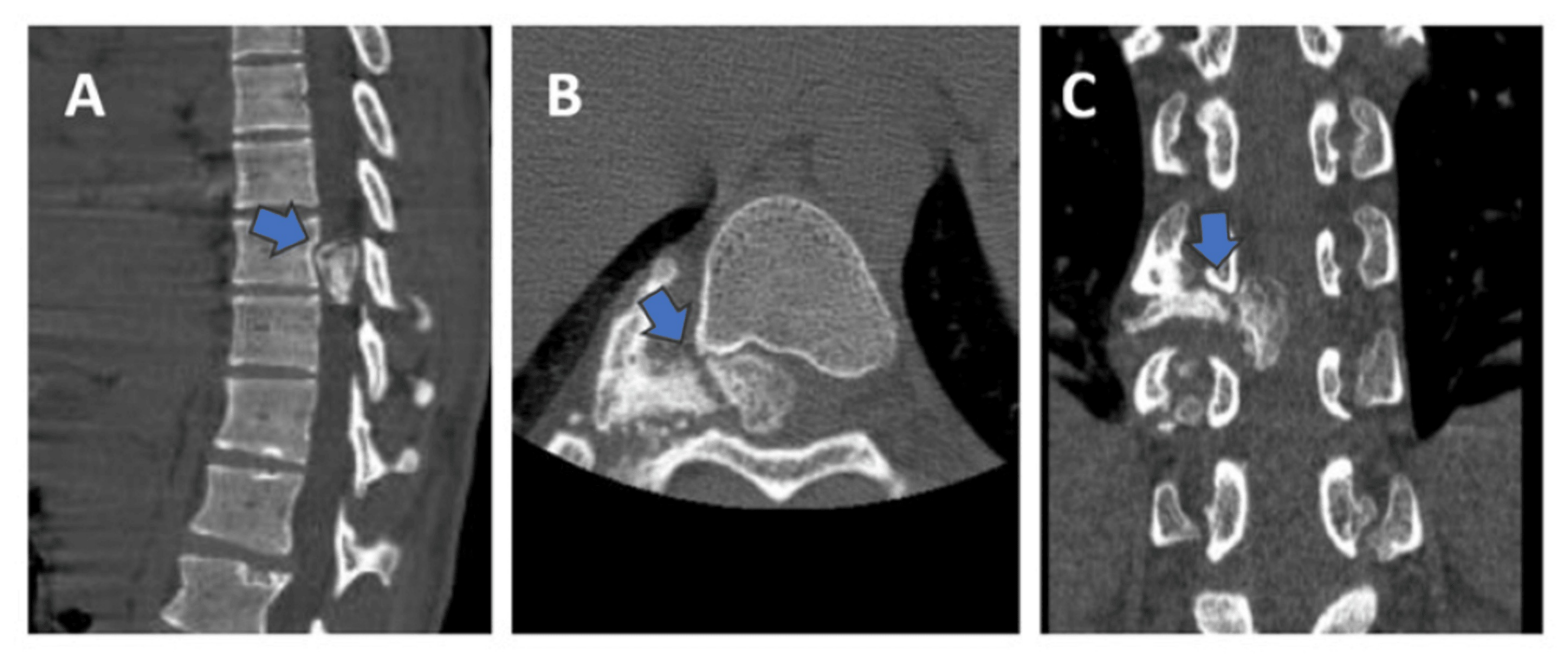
CT spine showing a bony mass arising from the posterior aspect of the ninth rib head extending into the D9-D10 neural foramen. (A) Sagittal section of CT spine showing a bony mass in the spinal canal and posterior scalloping of the D10 vertebra. (B and C) Axial and coronal sections of CT spine showing a bony mass arising from the posterior aspect of the ninth rib head extending into the D9-D10 neural foramen. CT: computed tomography.
